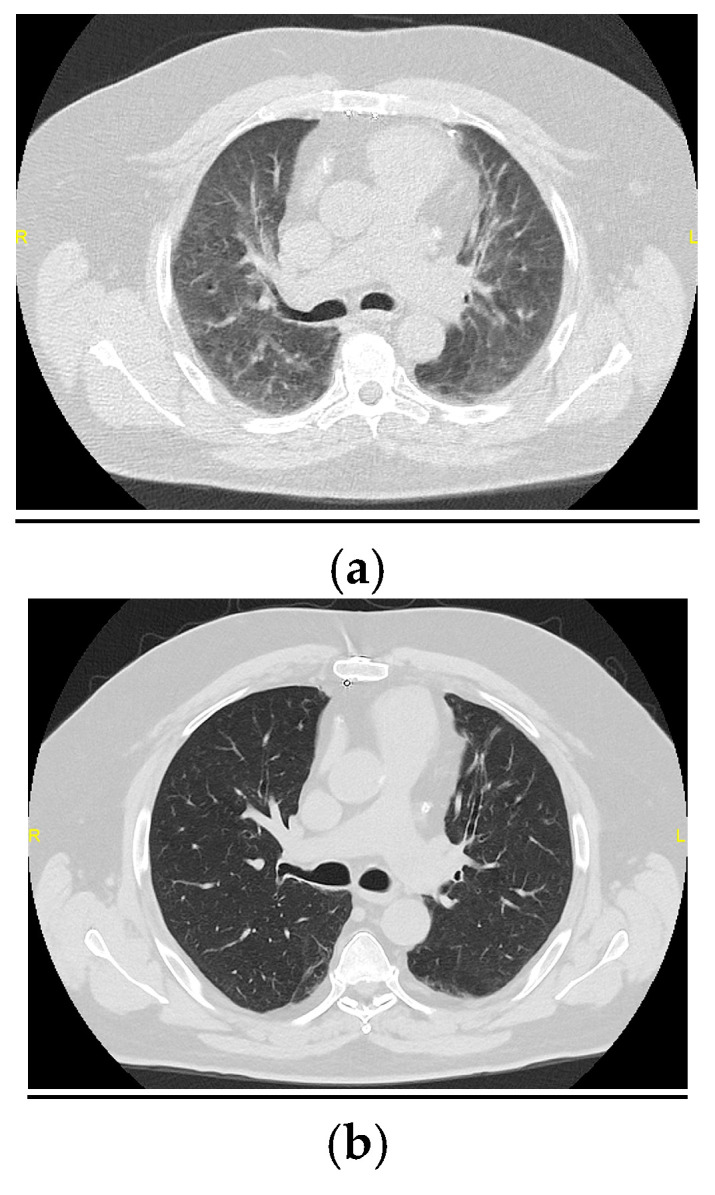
(a) HRCT image performed 6 months after hospital discharge showing diffuse ground-glass opacities and cylindrical bronchiectasis. (b) Image demonstrating complete resolution of the ground-glass opacities 12 months after hospital discharge, with the persistence of the bronchiectasis.
